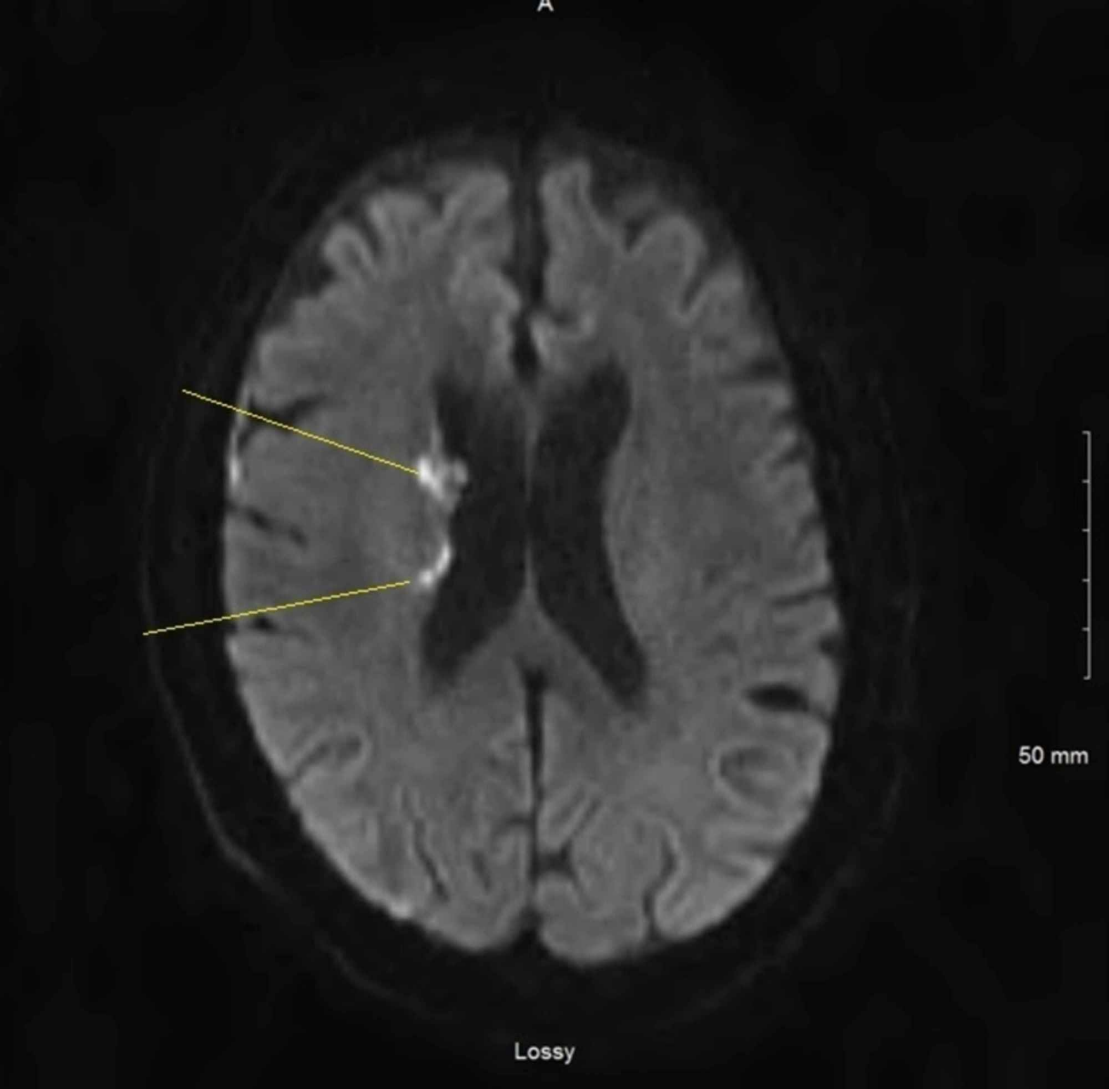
Magnetic resonance imaging of the brain showing patchy restricted diffusion and acute infarction.
